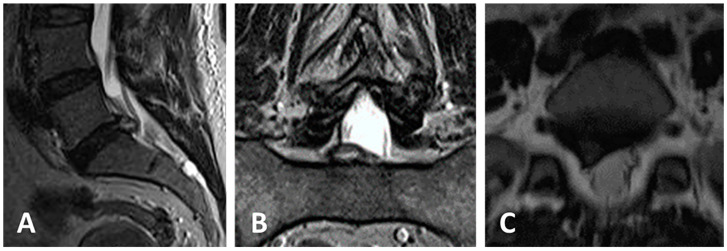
(A–C) Preoperative MRI shows an extruded disc herniation at the right L5-S1 level. VAS was 6, ODI was 56.
